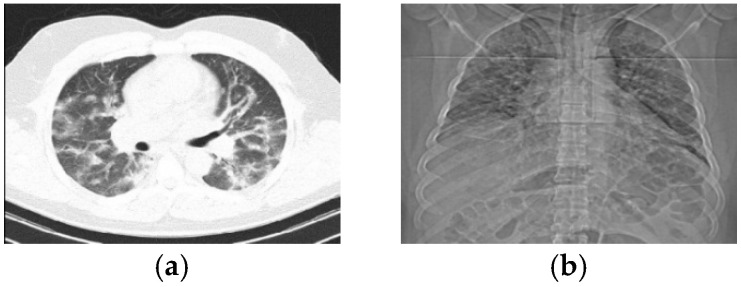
A lung image of a male person in two imaging types. (a) Lung CT-scan. (b) Chest radiography.
